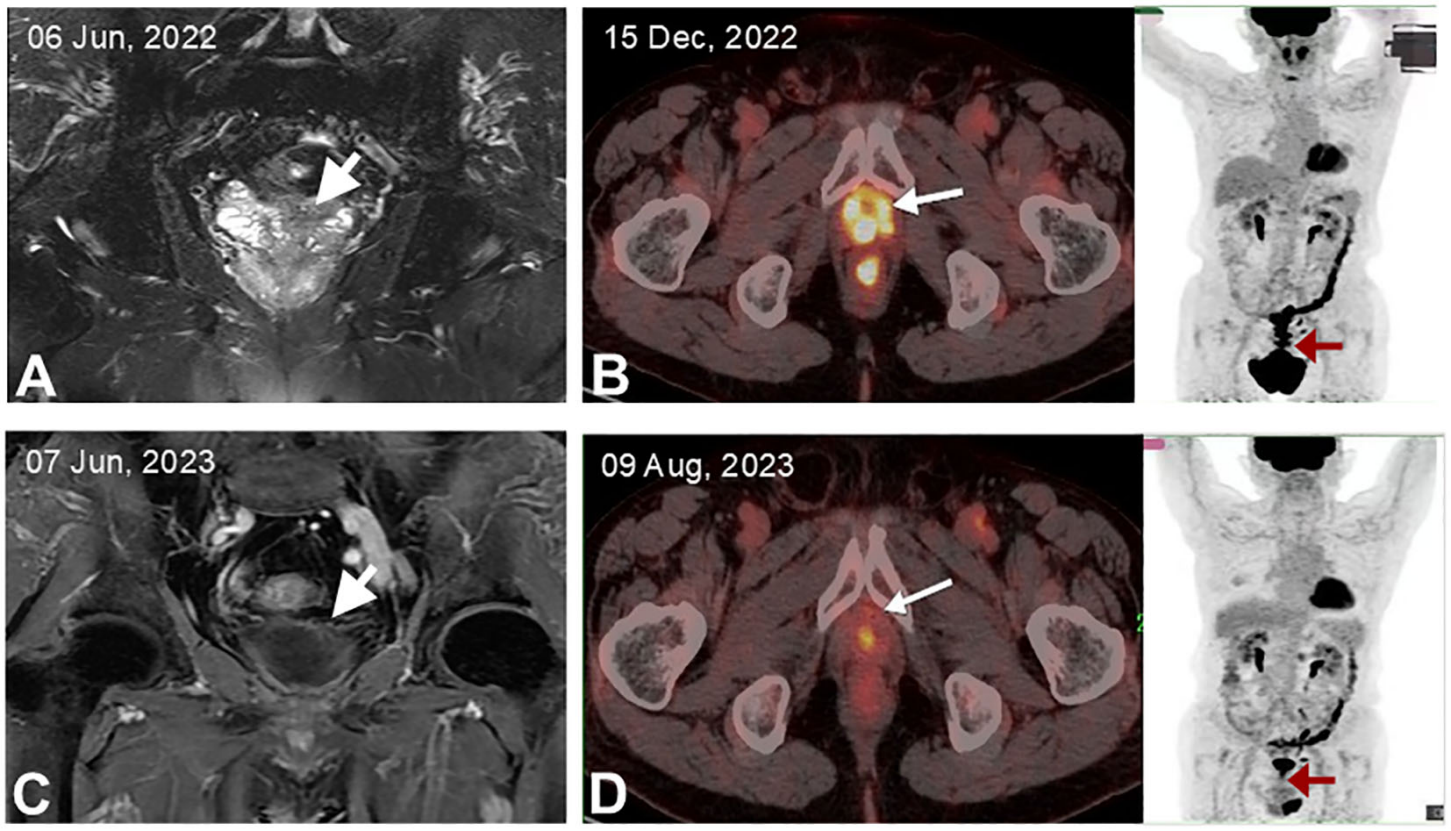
MRI and FDG-PET/CT imaging of the patient. (A) Initial MRI showing abnormal signals in the prostate and left seminal vesicle. (B) MRI after six cycles of R-CHOP treatment, indicating resolution of abnormal masses in the prostate and left seminal vesicle. (C) PET/CT post-radical prostatectomy, highlighting a maximum standardized uptake value (SUVmax) of 6.0 in the prostate fossa, with the arrow marking the specific loci. (D) PET/CT after completing six cycles of R-CHOP treatment, demonstrating a return to normal metabolic activity in the prostate fossa.
